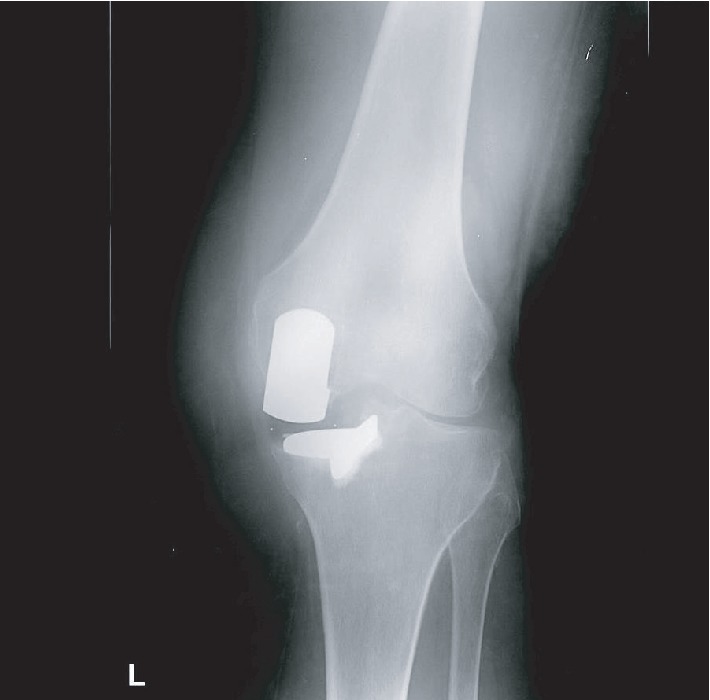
Weight-bearing AP radiograph of the left knee from nine months prior to presentation. Medial unicompartmental knee replacement in situ.
